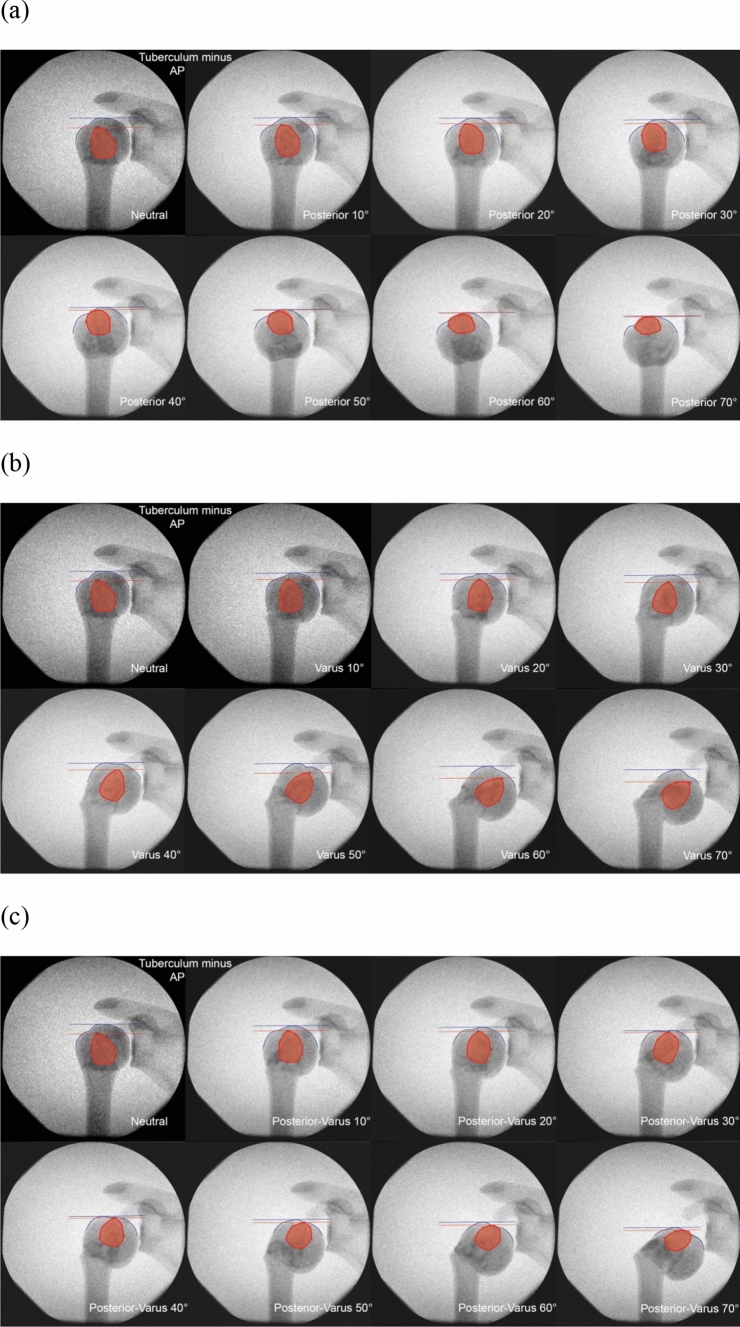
Anteroposterior view radiographic series of the lesser tuberosity (red) with increasing a posterior, b varus, and c combined posterior-varus angulation. Blue and red lines indicate the most proximal point on the head contour and tuberosity, respectively
